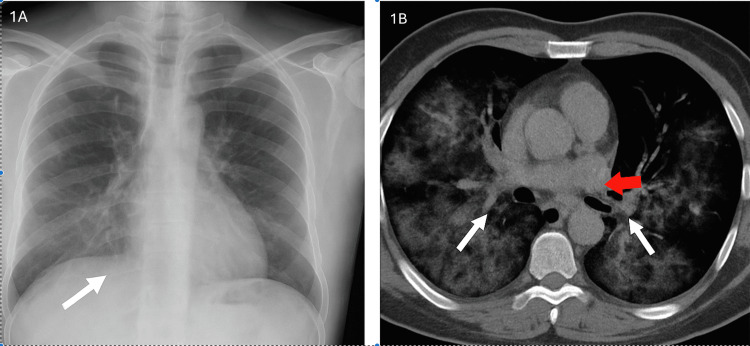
Imaging findings A. Chest X-ray demonstrates left mid-lower zone airspace shadowing (white arrow) consistent with possible consolidation. B. CTPA image reveals patchy bilateral ground-glass opacities (white arrows) and enlarged right hilar lymph node (red arrow). No evidence of pulmonary embolism is seen CTPA: computed tomography pulmonary angiogram
